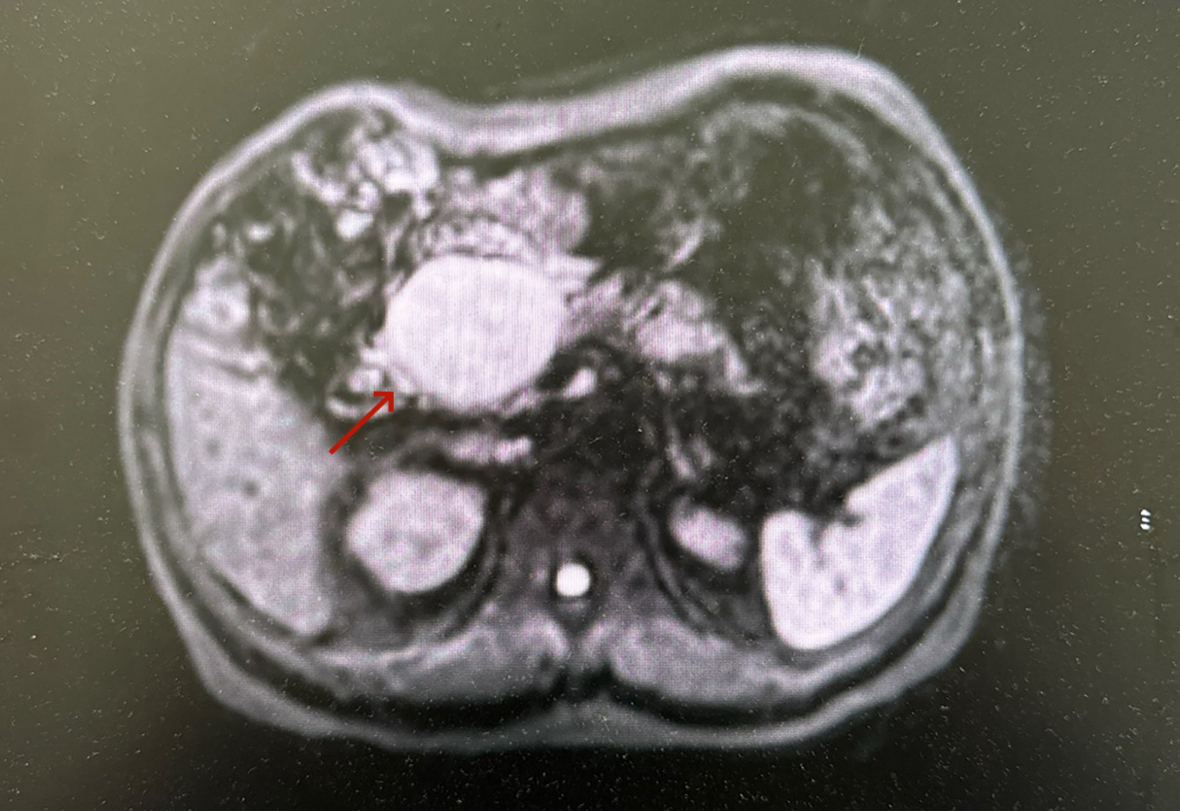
MRI showed a circular heterogeneous focal lesion on the pancreatic head, with a low signal on T1WI, and a high signal on T2WI and DWI. The lesion was approximately 50*58 mm in size, with uneven enhancement.
